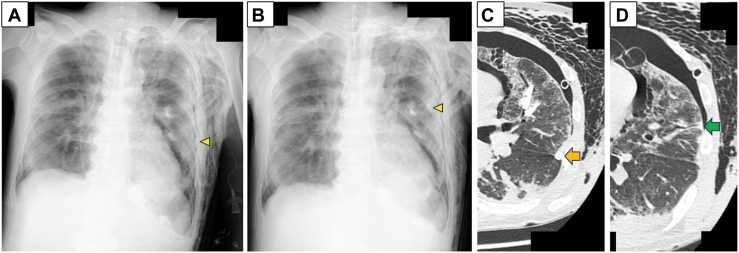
Chest radiographs on hospital days (A) 20 and (B) 21 after bronchoscopic intervention showing endobronchial Watanabe spigot displacement (arrowhead). (C) Chest computed tomography on hospital day 21 showing intrapleural migration of 1 spigot (arrow). (D) Corresponding axial image showing a tract extending from B5a to the visceral pleura (arrow).
